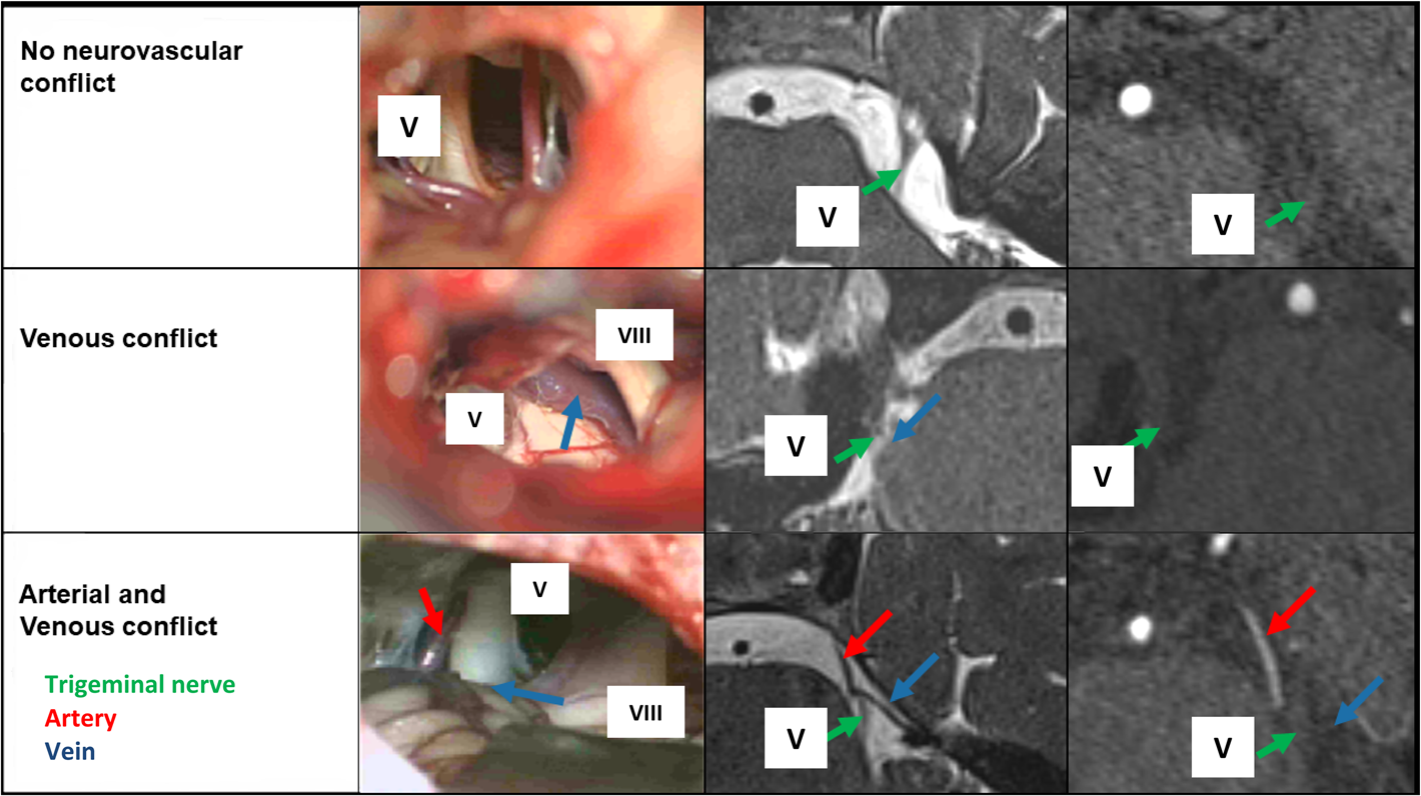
Comparison of microsurgical anatomy, MRI-SPACE and MRA-TOF (V: trigeminal nerve, VIII: vestibulocochlear nerve)
